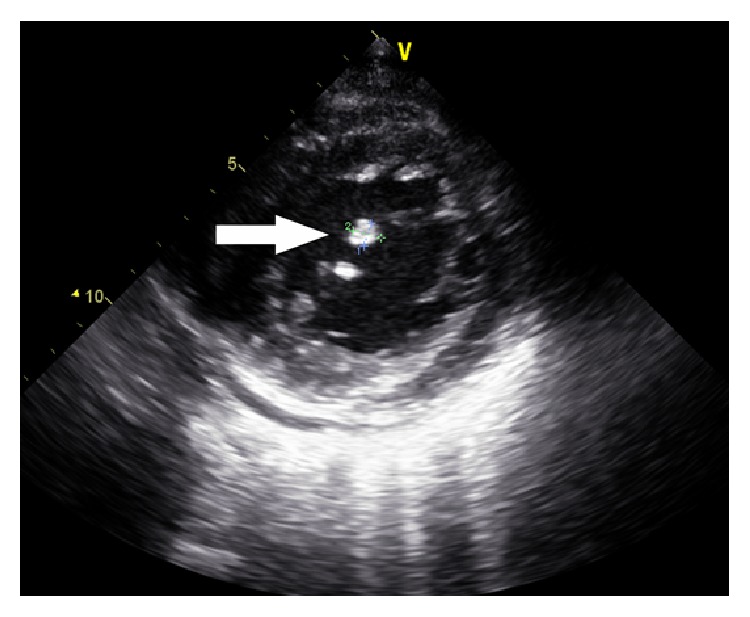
Echocardiography of the patient's showed vegetation on the mitral valve leaflet (7.67 mm*∗*6.14 mm), mitral valve prolapse and moderate regurgitation, mild degree backstreaming of tricuspid valve, and left ventricular enlargement.
